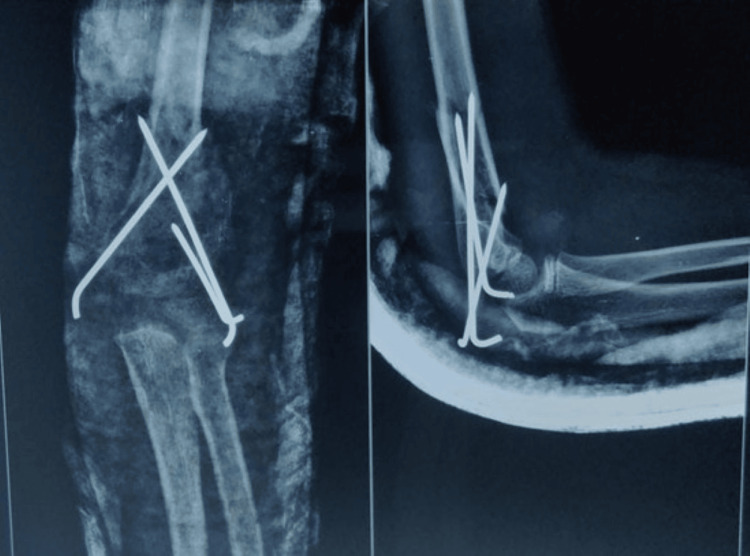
Postoperative radiograph of anteroposterior and lateral views, showing anatomical reduction of the fracture and proper placement of the pins.
